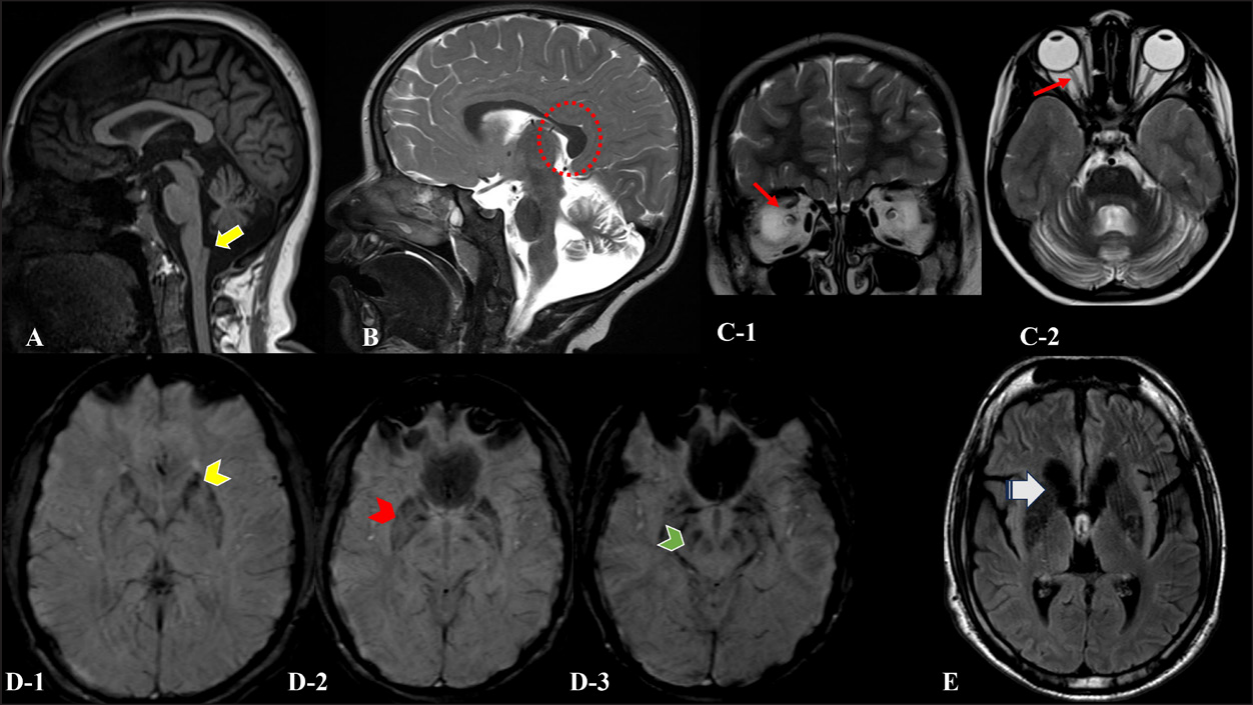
Magnetic resonance imaging of the brain of selected patients. A: Patient of early-onset parkinsonism (Patient-22). T1 mid-sagittal image showing claval (gracile tubercle) hypertrophy (yellow arrow). B: Patient of infantile neuroaxonal dystrophy (Patient-12). T2 mid-sagittal image showing vertically oriented splenium of the corpus callosum (red dotted circle). C: Patient of atypical neuroaxonal dystrophy (Patient-16). T2 coronal (C-1) and axial (C-2) images showing bilateral optic nerve atrophy (red arrow). D: Patient of complicated hereditary spastic paraparesis (Patient-13). SWI image showing mineralization of bilateral caudate (D-1, yellow arrowhead), putamen, globus pallidi (D-2, red arrow head), and substantia nigra (D-3, green arrow head). E: Patient of complicated hereditary spastic paraparesis (Patient-19). Fluid attenuated inversion recovery (FLAIR) axial image showing atrophy of bilateral caudate and generalized cerebral atrophy (white arrow). It also shows FLAIR hypointensity of bilateral putamen and globus pallidi.
